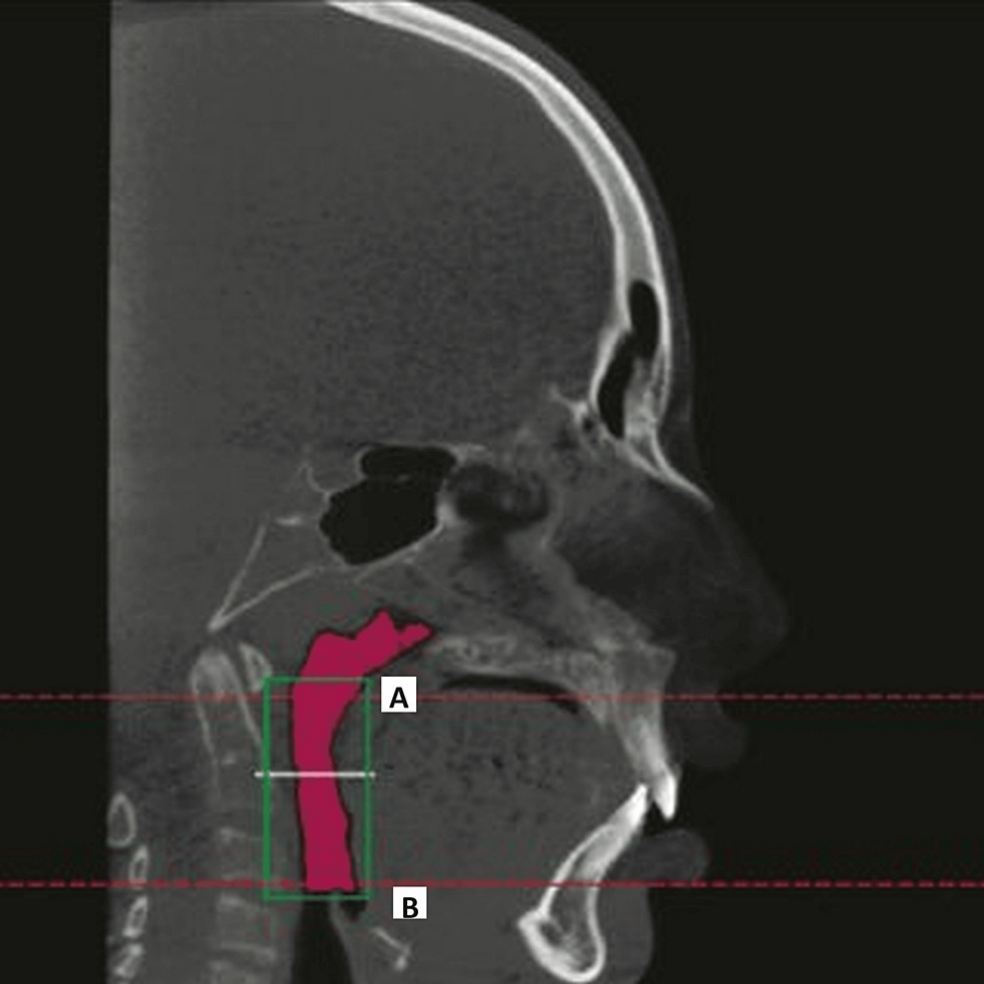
CBCT depicting the pharyngeal space with the area of interest A: superior limit B: inferior limit
